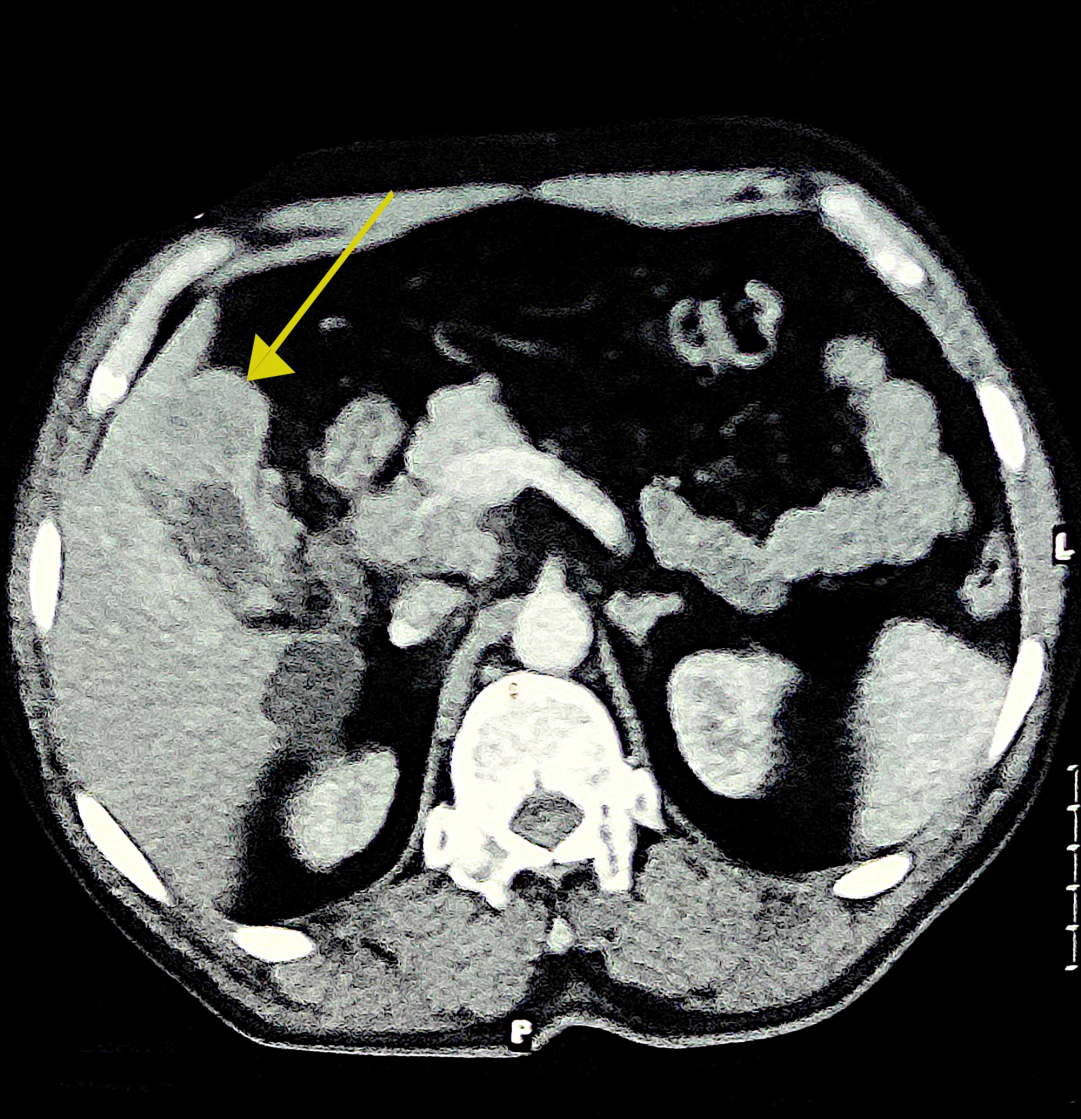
Contrast-enhanced CT scan of the abdomen showing gallbladder mass with infiltration into liver
